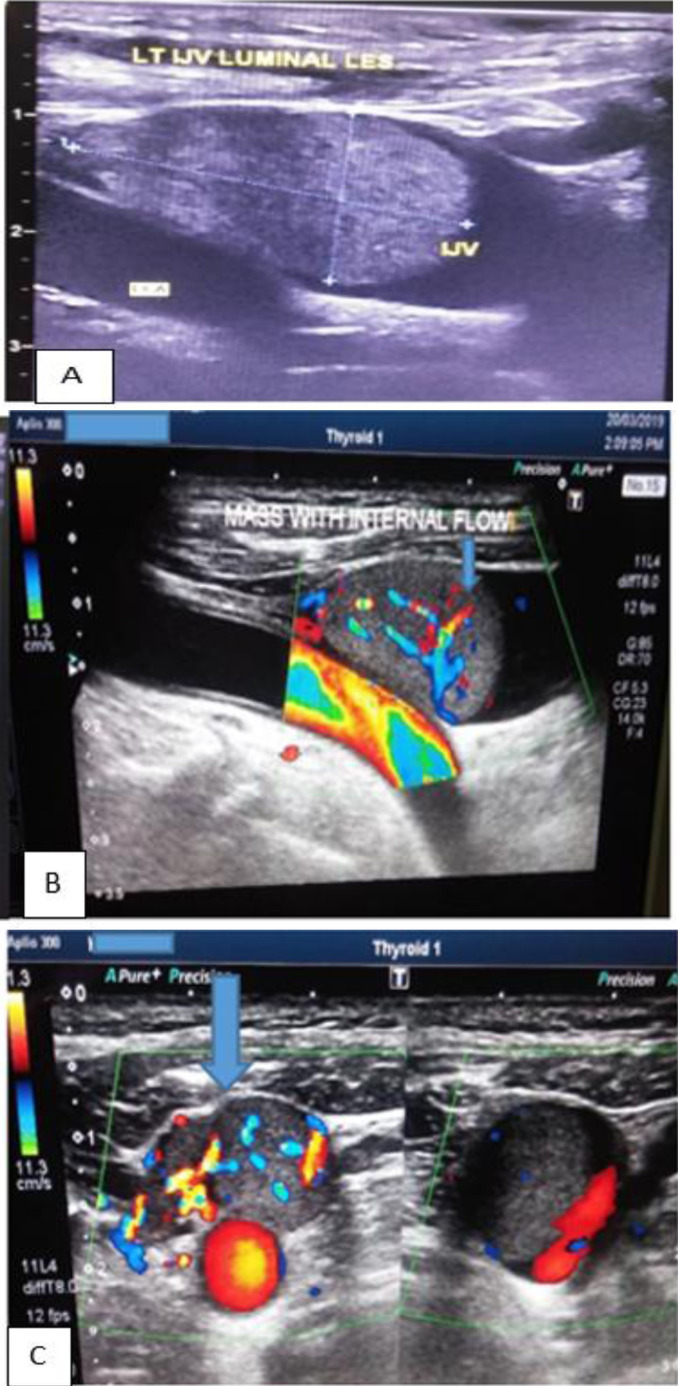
Grayscale (A) and colour Doppler ultrasound images (B and C) of the neck showing homogeneous left IJV intraluminal lesion (A) and significant internal vascularity (B & C).
